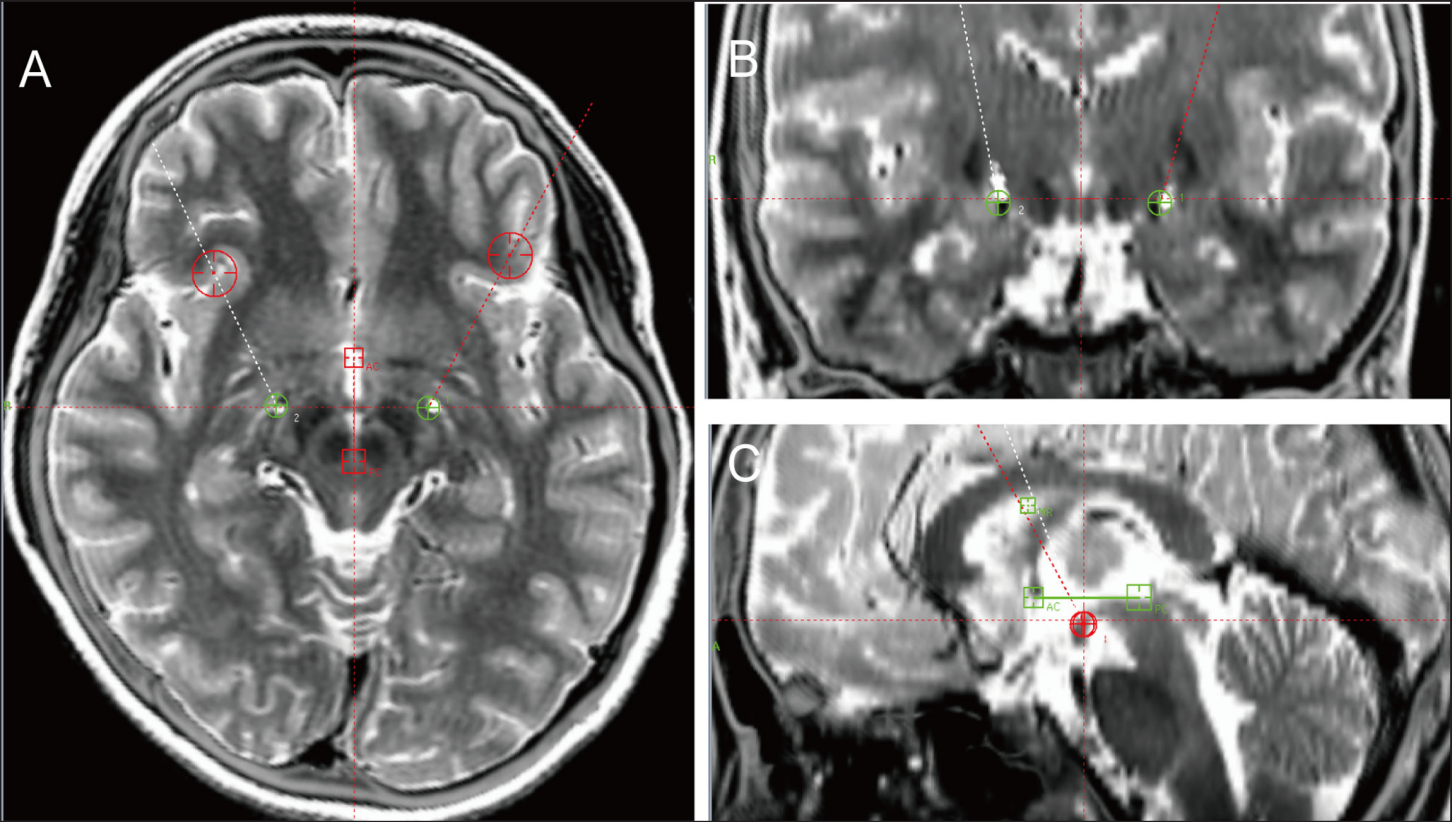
Postoperative CT images fused with preoperative MR images demonstrating the positions of the implanted electrodes in GPi (1A, 1B, 1C). The two red orthogonal vertical lines refer to the Cartesian coordinate system in each view, while the two slashes, with or without red circles, present the trajectories of the implanted leads. In the center of each view, two green or red circles show the planned targets. AC, anterior commissure; PC, posterior commissure; MR, midline reference.
